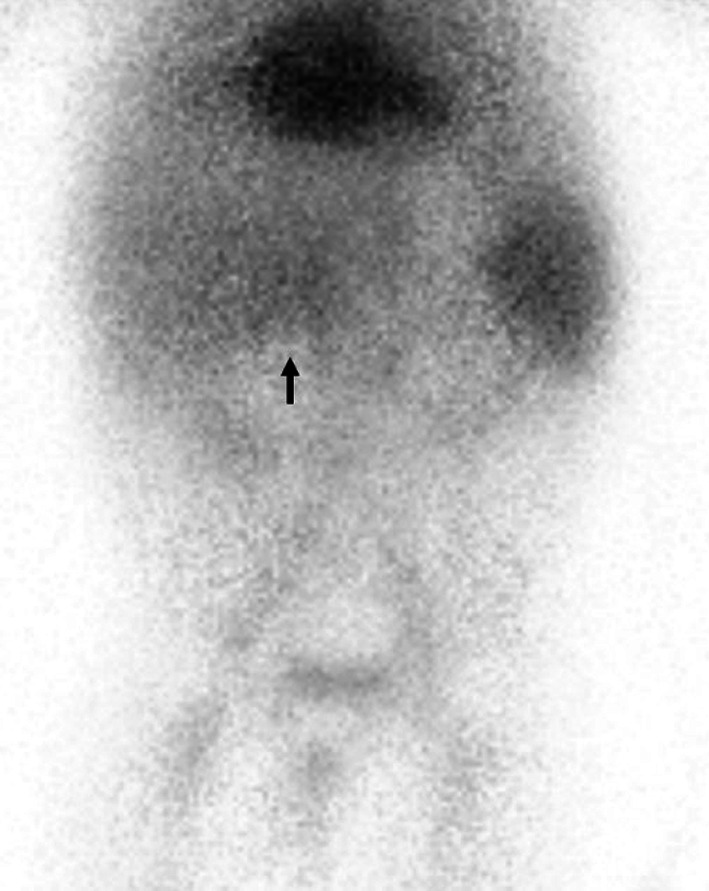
Hemorrhage scintigraphy. Technetium-99 m-human serum albumin-diethylenetriaminepenta-acetic acid (740 MBq) was administered intravenously. The concentration at the portoenterostomy site (arrow) peaked 24 min after injection. After 60 min, the concentration did not migrate
